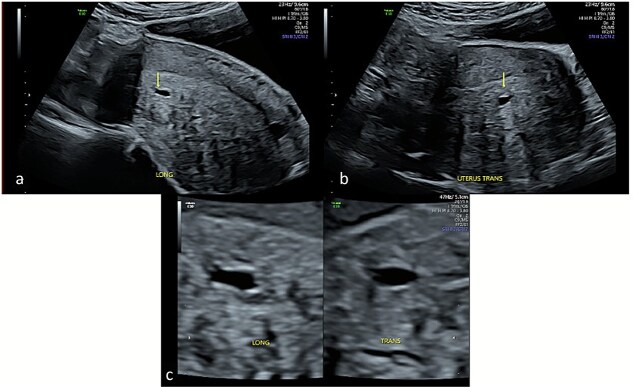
Transabdominal ultrasound of the uterus demonstrating cystic sub-endometrial spaces that were mistaken for a gestational sac in earlier ultrasound scans. The sub-endometrial space is demonstrated in longitudinal (a) and transverse planes (b) and under magnification (c) with a notable absence of decidual reaction around the cystic space.
